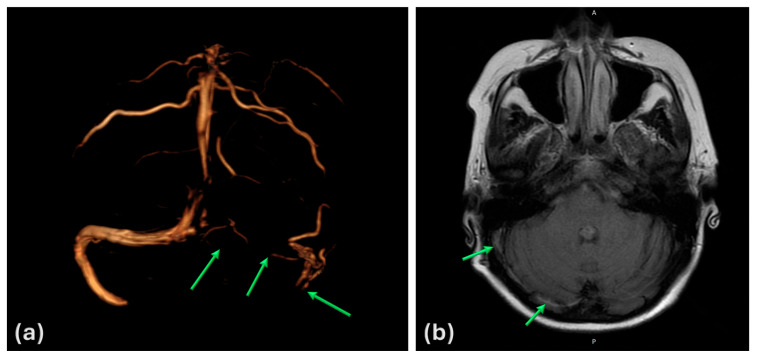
The brain MRI performed the following day revealed thrombosis in the right transverse and sigmoid sinuses on the venous angiography sequence 2D TOF ((a), arrows) and reduced flow in these sinuses on the FLAIR sequence ((b), arrows).
